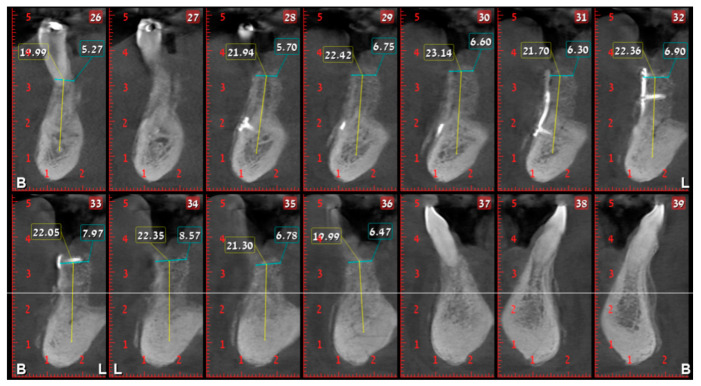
Alveolar ridge bone volume 4 months following reconstruction in CBCT imaging; cross-sections of reconstruction.
